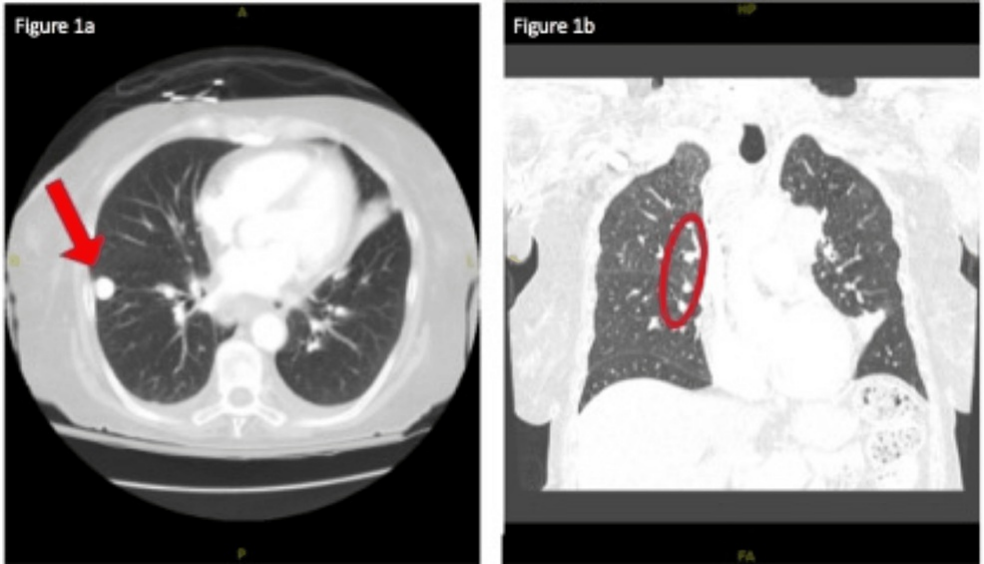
CT chest with contrast. (a) Right-sided coin lesion shown by the red arrow. (b) Mediastinal and left and right hilar adenopathy. Right-sided adenopathy is shown in the red circle.
